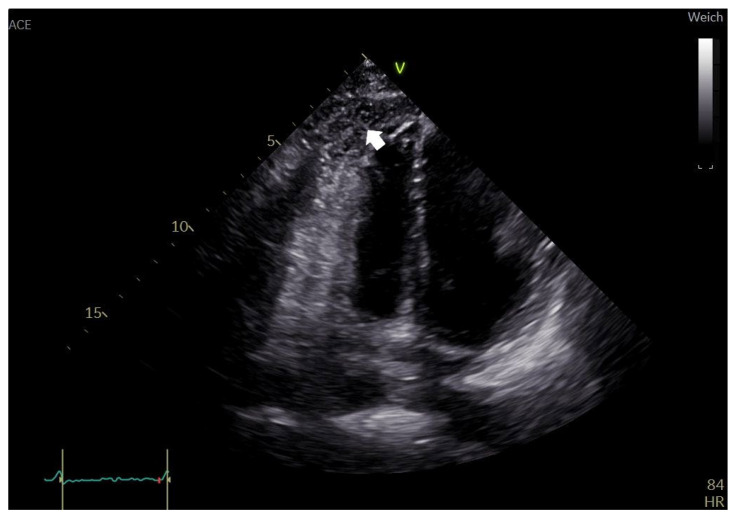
Transthoracic echocardiography apical four-chamber view showing small echogenic spots (arrow) swirling within the pericardial cavity.
